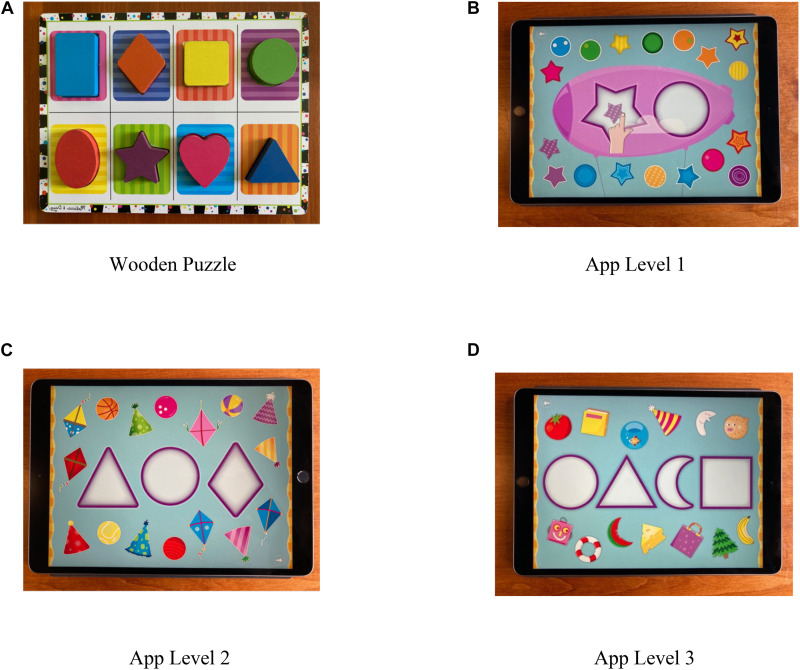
Wooden (A) and electronic (B–D) shape matching puzzles. The wood puzzle is from the *Melissa and Doug Toy Company*, Wilton, CT, United States. The electronic puzzles are apps from TinyHands, tinyhandsapps.com.
